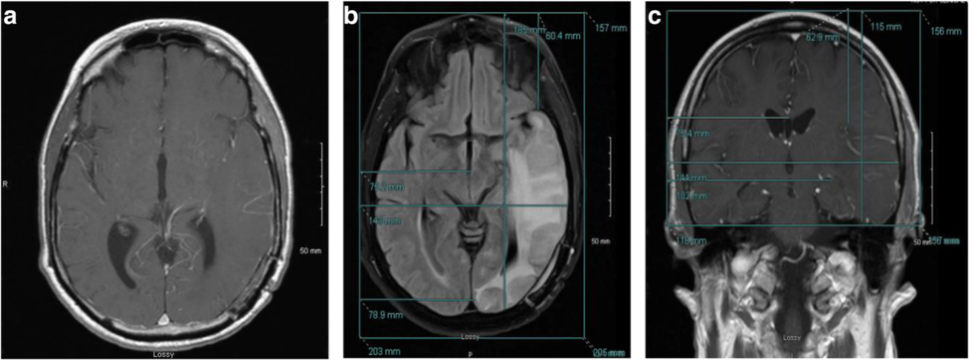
a, b & c: Figure a demonstrates lack of enhancing disease on an axial T1 postcontrast scan in a glioblastoma patient experiencing disease progression following 5 months of treatment with bevacizumab. Figures b shows the corresponding slice on the axial FLAIR sequence demonstrating significant FLAIR signal abnormality. As such, TTFields planning was performed on the FLAIR sequence. Measurements were obtained from a reference frame drawn at the level of the scalp in a standard manner, and measuring from the right frame to the near and far borders of the FLAIR signal abnormality. Figure c depicts mapping performed in the coronal plane to a corresponding area of hypodensity on the T1 postcontrast sequence. All measurements are obtained from a reference frame drawn at the level of the scalp, extending inferiorly to the lower margin of temporal lobe
